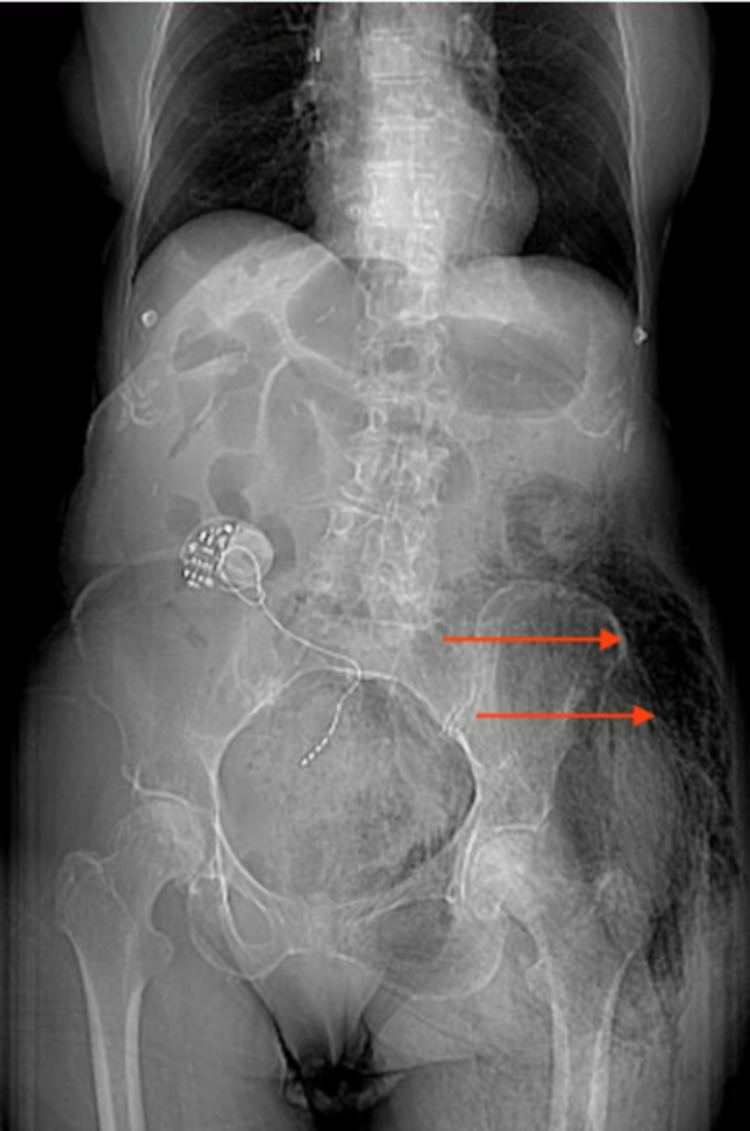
Computerized tomography (CT) abdomen and pelvis with contrast anteroposterior view showing free air in left gluteal and hip regions (red arrows)
